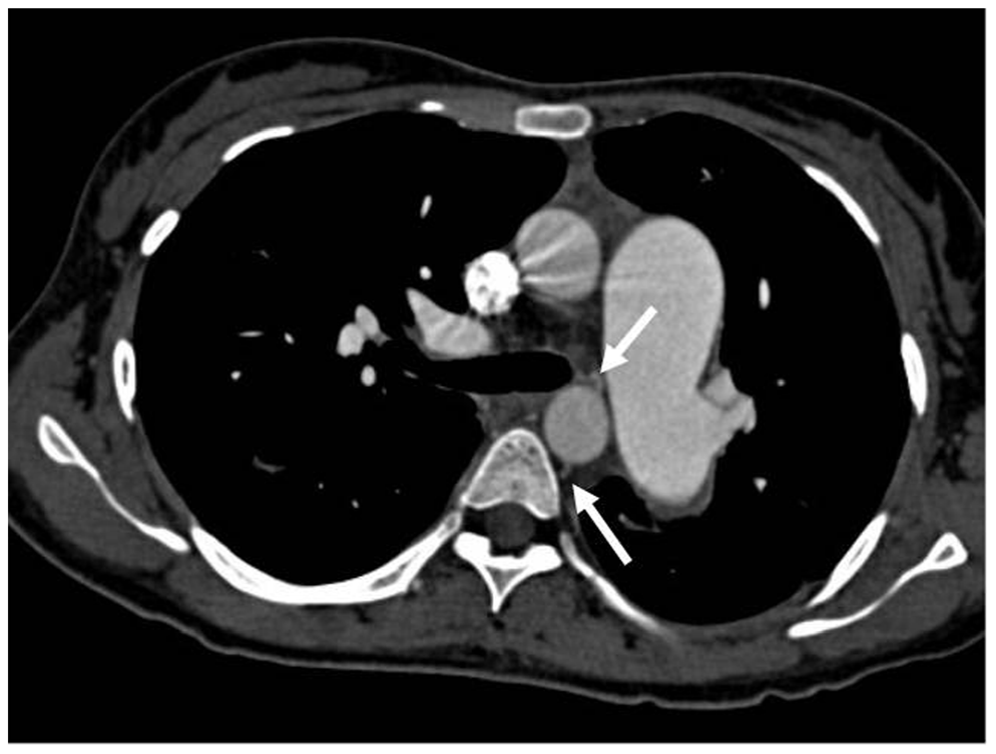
Shown is a CT angiography scan of a 23 year old iPAH patient with presence of bronchial artery hypertrophy. (upper arrow: left bronchial artery; lower arrow: right bronchial artery).
